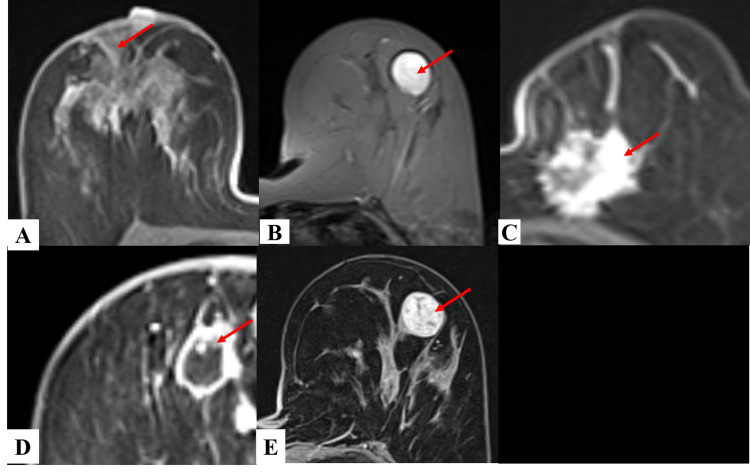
Five patterns of post-contrast enhancement of breast lesions on contrast-enhanced T1 fat-saturated MR images (A) non-mass-like enhancement, (B) homogenous enhancement, (C) heterogeneous enhancement, (D) rim enhancement, (E) enhancement of the lesion with dark internal septations
